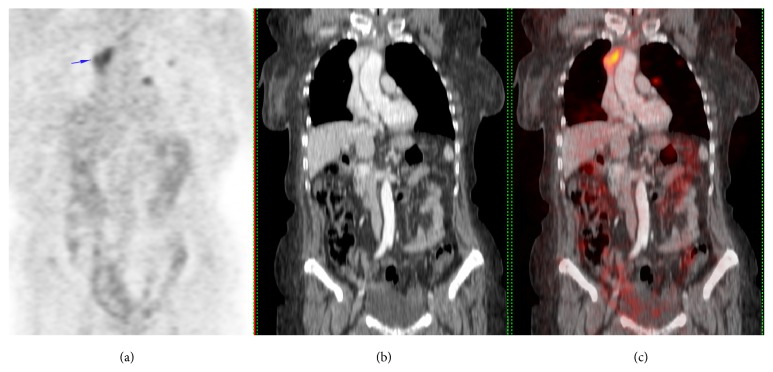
(a) An uptake in the 18F-fluoro-D-glucose PET and (b) small depletion defect in the innominate vein growing towards the superior cava vein, and when we did the fusion with CT scan, (c) intravascular metastasis was found.
